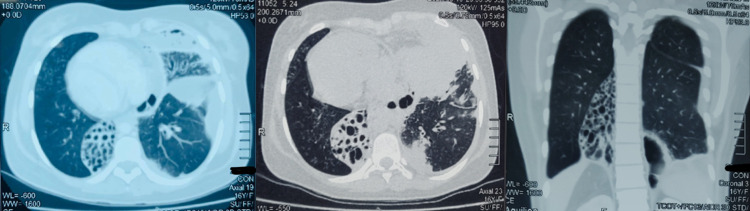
HRCT A (left): Axial view showing right sided cystic bronchiectasis with left sided empyema. B (center): Axial view showing similar finding with empyema extending to anterior mediastinum. C (right): Coronal view showing right sided bronchiectasis with left sided empyema obliterating the costophrenic angle. Note dextrocardia in A and B. HRCT, high resolution computed tomography
